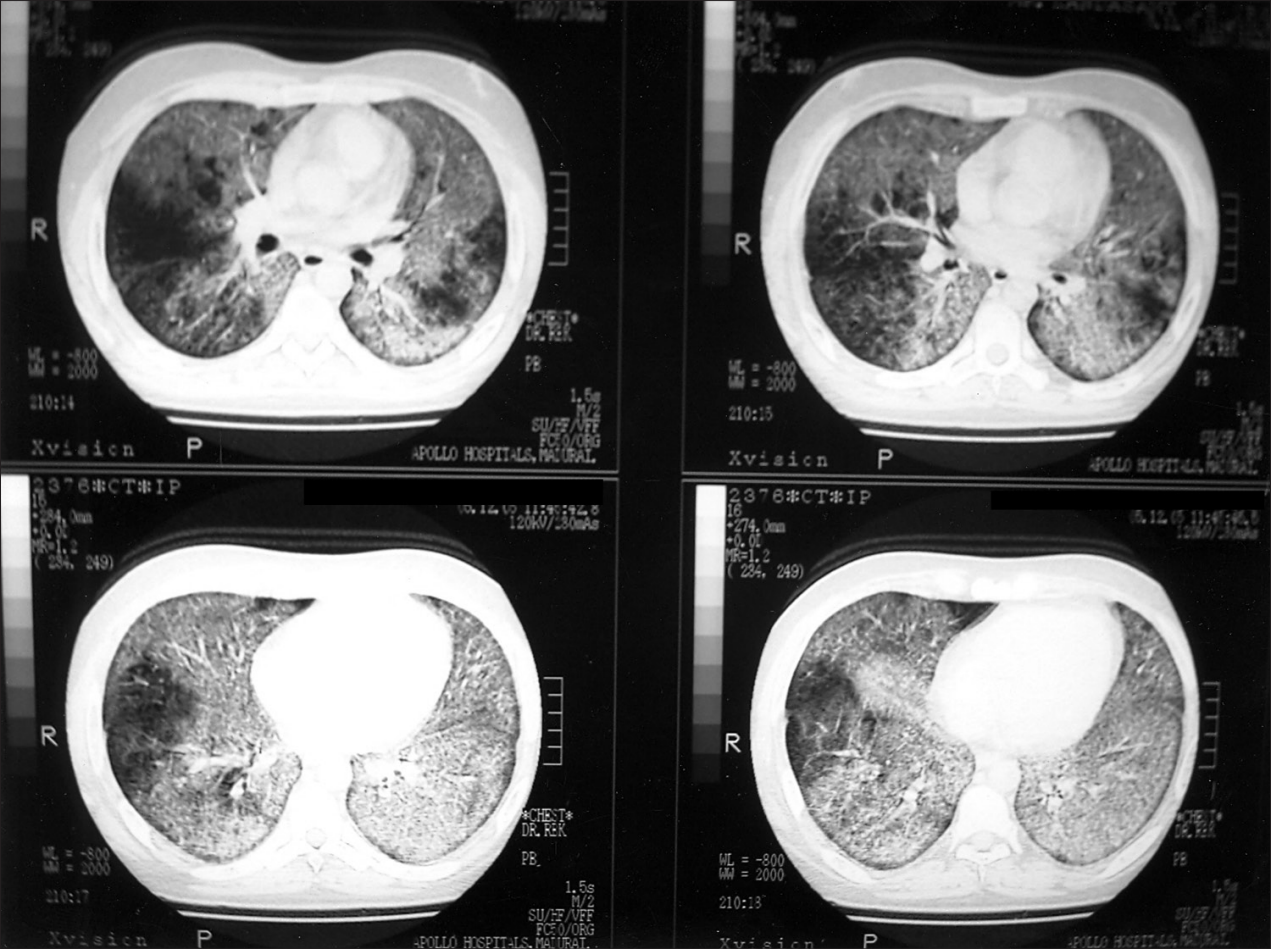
CT chest showing bilateral ground glass, “Crazy Paving” pattern
